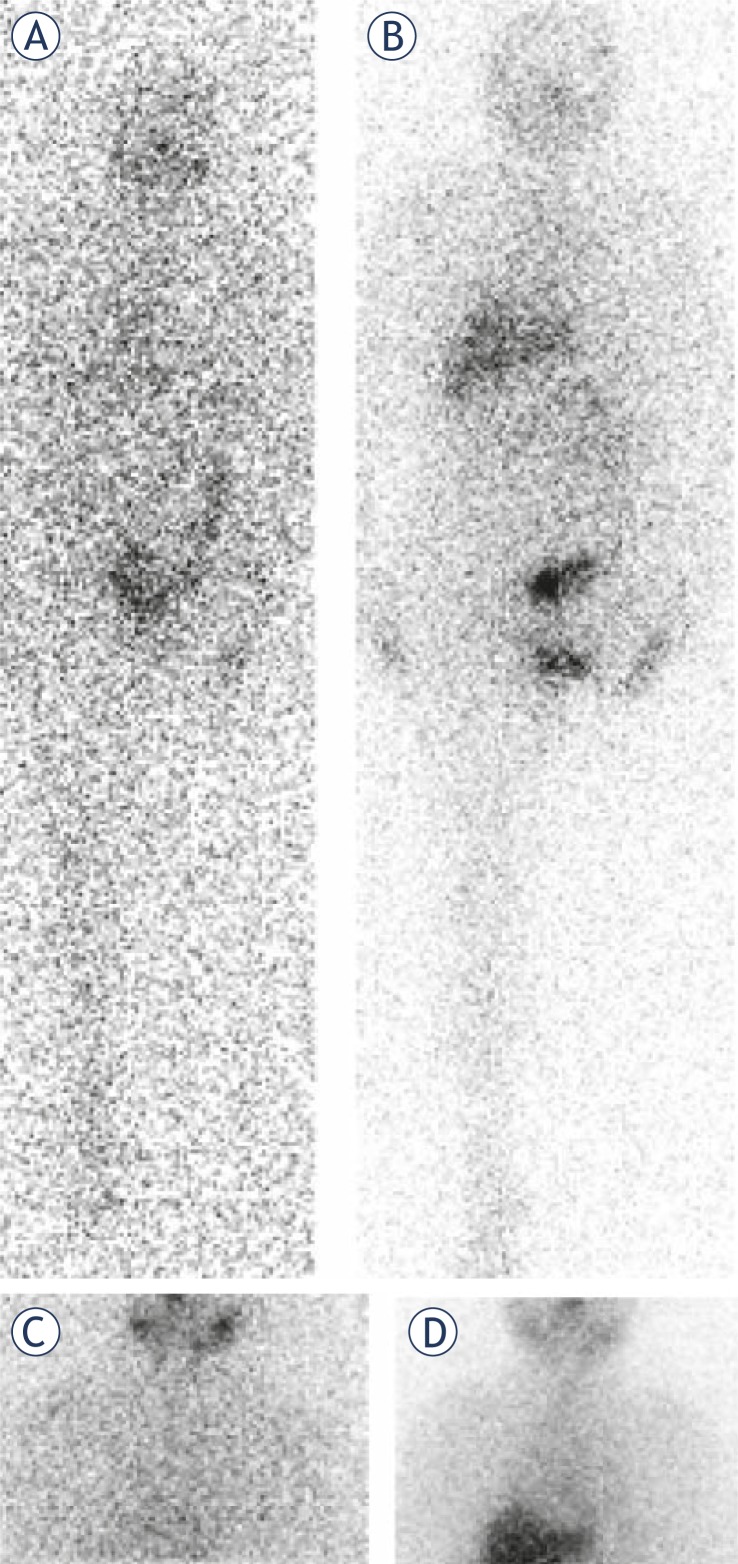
Radioiodine scans in the patient who underwent therapy with 7400 MBq (200 mCi) of ^131^I. This patient had small pulmonary nodules seen on computed tomography images performed 7 months before LID. A diffuse radioiodine uptake is seen in liver. Focal accumulation of ^131^I was not visible. Prior to LID, this patient had urinary iodine concentration of 169.6 μg/L, and post-LID value was 89.0 μg/L. Anterior whole body scans (A) prior to LID and (B) post LID; static scans of the neck and the thorax region (C) prior to LID and (D) post LID.
